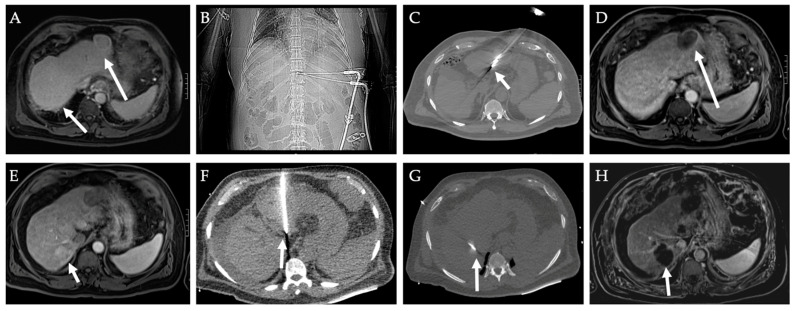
Microwave ablation and irreversible electroporation of hepatocellular carcinoma. A 72-year-old male with alcohol-induced cirrhosis and HCC was found to have a 3.5 × 2.6 cm biopsy-proven HCC lesion (white arrow) in segment II (A), LI-RADS 4 lesion in segment VII, and LI-RADS 3 lesion in the caudate lobe on MRI. (B,C) The patient underwent percutaneous MWA of the segment II HCC. (D) Four-month follow-up MRI showed complete response of the segment II HCC to MWA, but the two previously noted lesions (E) in the caudate lobe and segment VII progressed to LI-RADS 5. The patient underwent image-guided percutaneous ablation of these two lesions two months later, receiving (F) NanoKnife IRE for segment II/caudate and (G) MWA for segment VII lesion. (H) One-month follow-up MRI shows no residual tumor. The patient was transplanted two months later.
